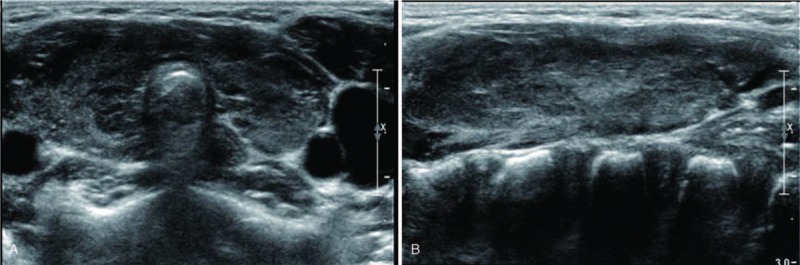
(A) Transverse and (B) longitudinal ultrasonographic images of Grade 3.
